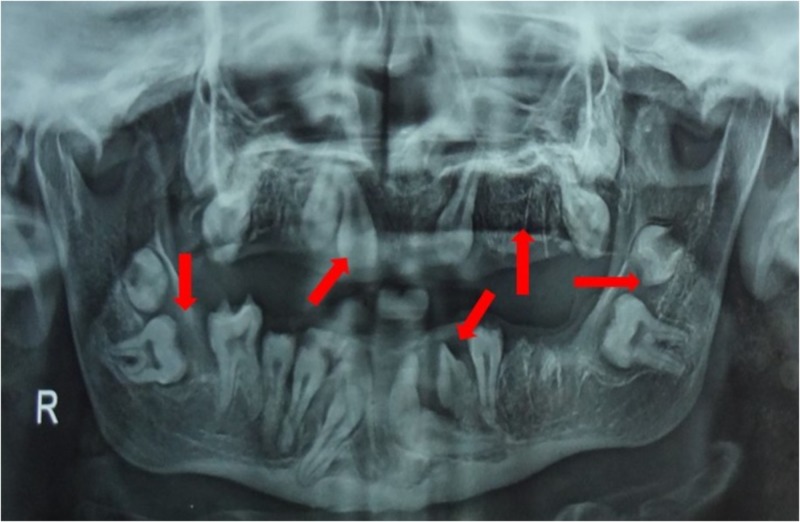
Pre-operative orthopantomogram. Preoperative radiograph demonstrating multiple impacted teeth in both maxilla and mandible, extraction sockets in the maxilla bilaterally and in the mandible on left side.
